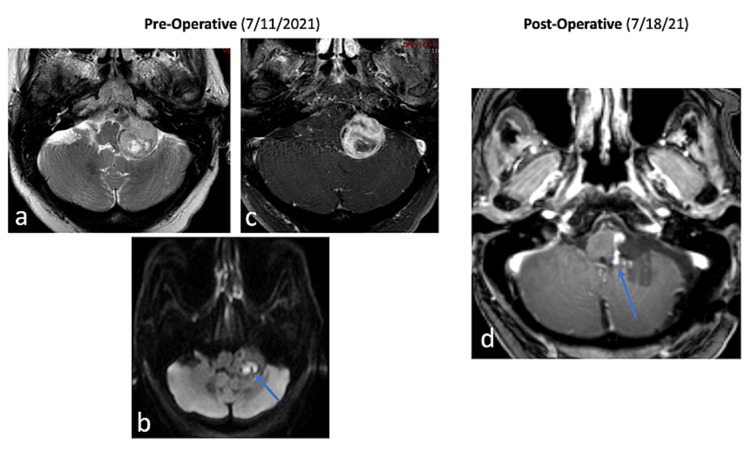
Pre- and postoperative imaging Preoperative images are shown in panels a-c and postoperative images in panel d. (a) Axial T2-weighted image demonstrates heterogeneous mass with an area of central T2 hyperintense signal due to cystic/necrotic changes. (b) Axial diffusion-weighted imaging (DWI) demonstrates restricted diffusion within the tumor (blue arrow on DWI). (c) Axial T1-weighted post-contrast image demonstrates prominent solid peripheral enhancement with central non-enhancement due to cystic/necrotic changes. (d) Postoperative MRI axial T1-weighted post-contrast image demonstrates resection of the mass with small residual nodular enhancing tumor along the medial margin (arrow) MRI: magnetic resonance imaging
